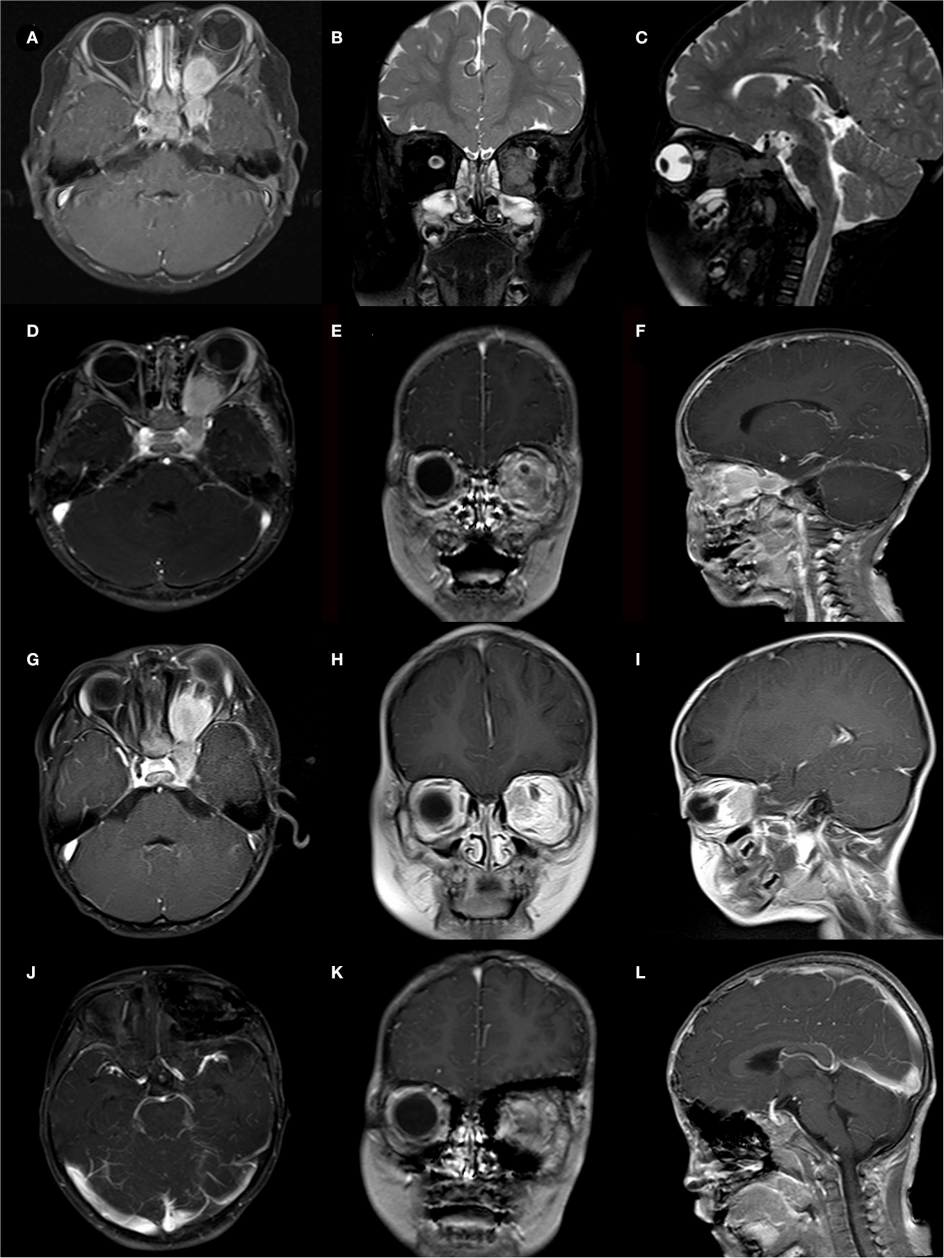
A case of 2-year-old boy presented with a cranial-orbital lesion. (A–C) Preoperative MRI showed a left dumbbell-shaped lesion with invasion of extraocular muscles and cavernous sinus. (D–F) STR was conducted to remove lesions about 1 cm^*^1 cm from the orbital apex, and pathological biopsy reveals a WHO-grade-I meningioma. (G–I) MRI follow-up at 12 months showed tumor progression and the second surgery was taken. (J–L) MRI follow-up at 18 months after the second surgery showed no tumor recurrence but the patient suffered severe visual failures.
